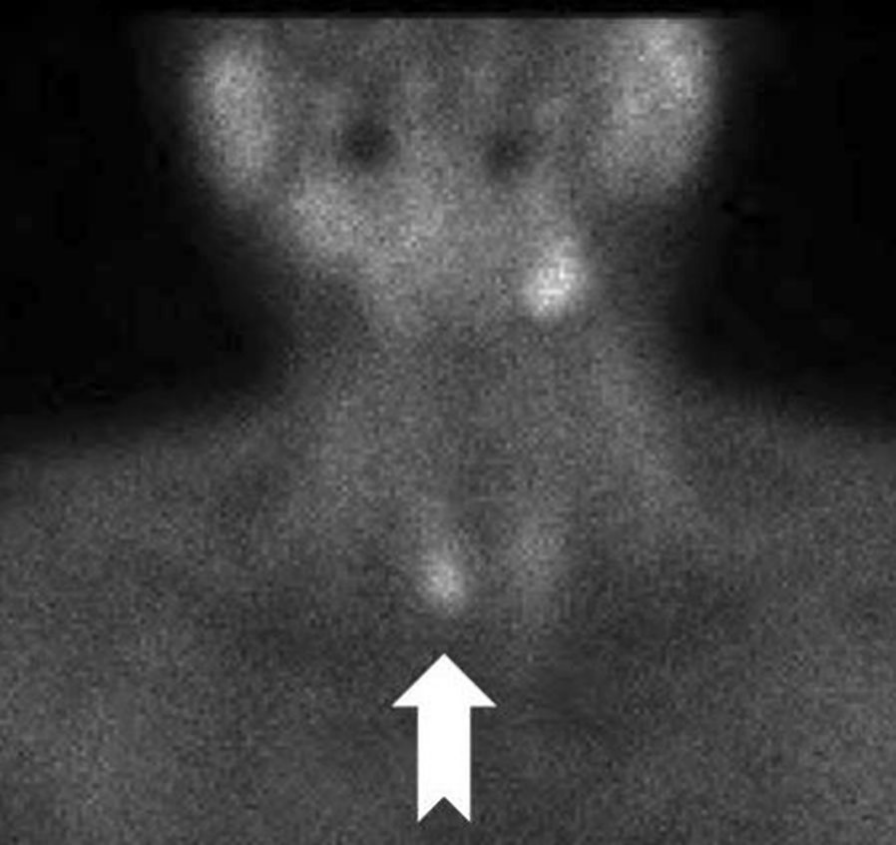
MIBI scintigraphy The MIBI scintigraphy showed accumulation near the lower right pole and strong accumulation even in the delayed phase, suggestive of a parathyroid adenoma
